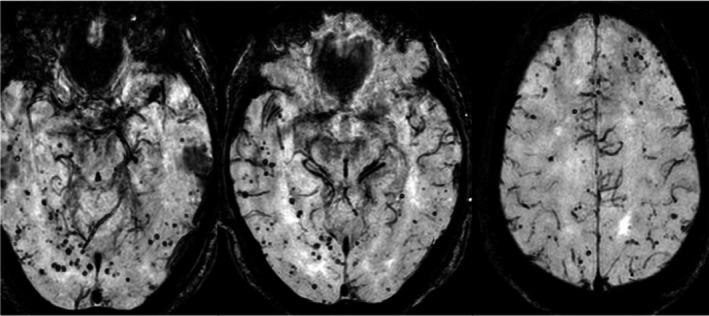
MRI scan showed cerebral amyloid angiopathy, several microbleeds in the infra‐ as well extratentorial region. A 67 years patients presented with increasing cognitive impairment in the last year
